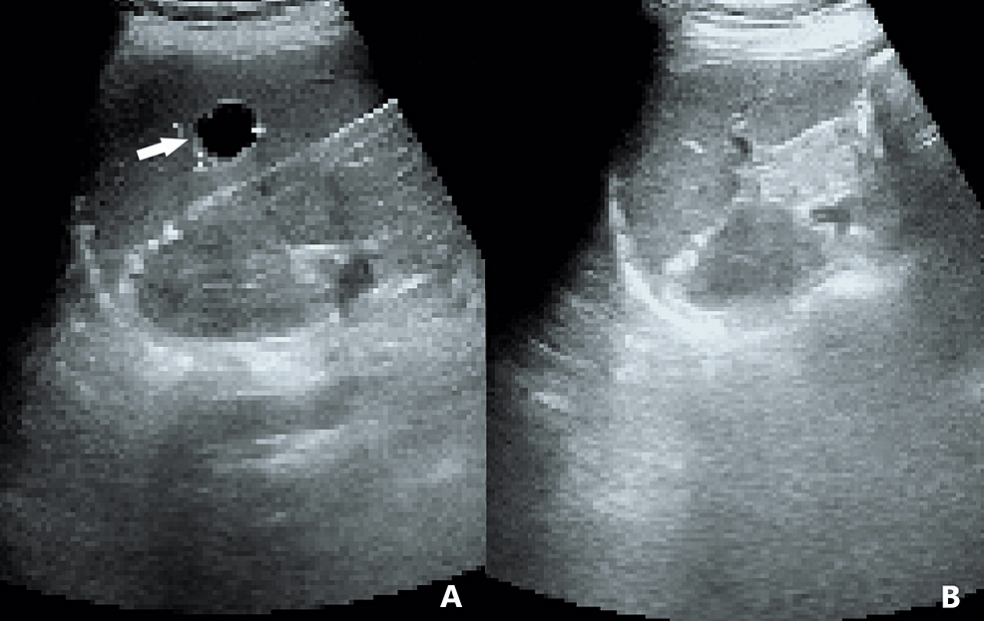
Ultrasound scans of the spleen of the patient before and after dietary intervention. (A) Sonogram showing a splenic cyst (arrow) measuring 18.9 mm x 17 mm in year one. (B) No cyst can be seen on the ultrasound scan in year six after two courses of dietary intervention, which were carried out in year three and year six, respectively.
